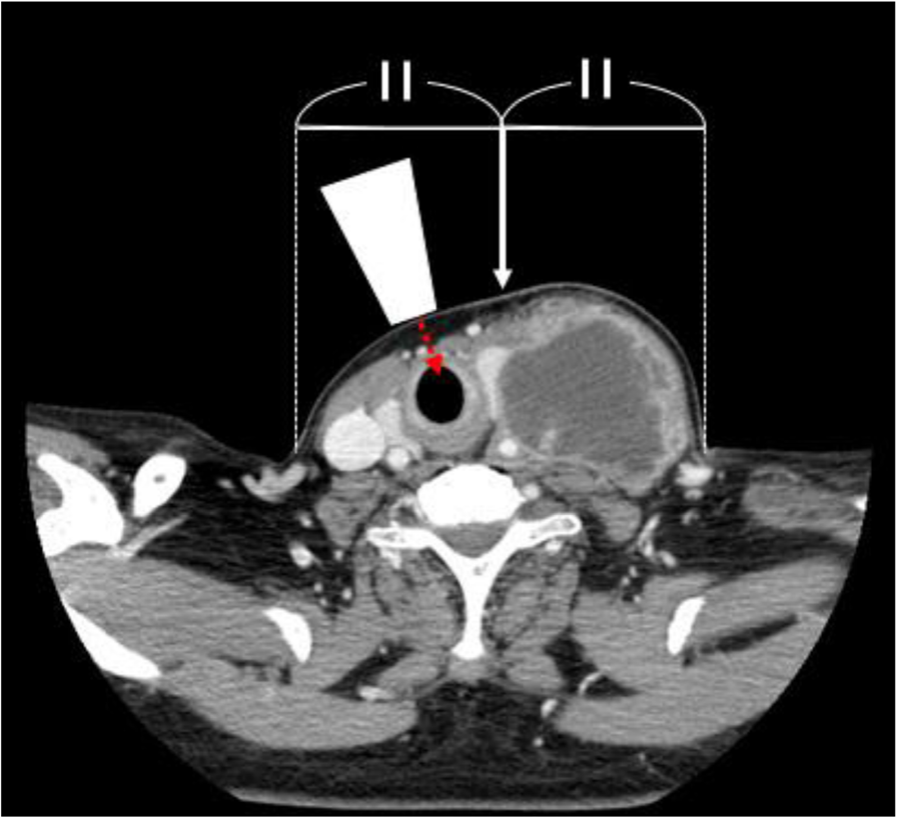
Cervical computed tomography The white arrow indicates the apparent center of the neck. The true center (sagittal line) of the neck is present toward the right side. The ultrasound probe (white trapezoid) is placed perpendicularly to the skin and the ultrasound beam (red dashed arrow) directed to the cricothyroid membrane. The trachea is deviated to the right and rotated to the right
